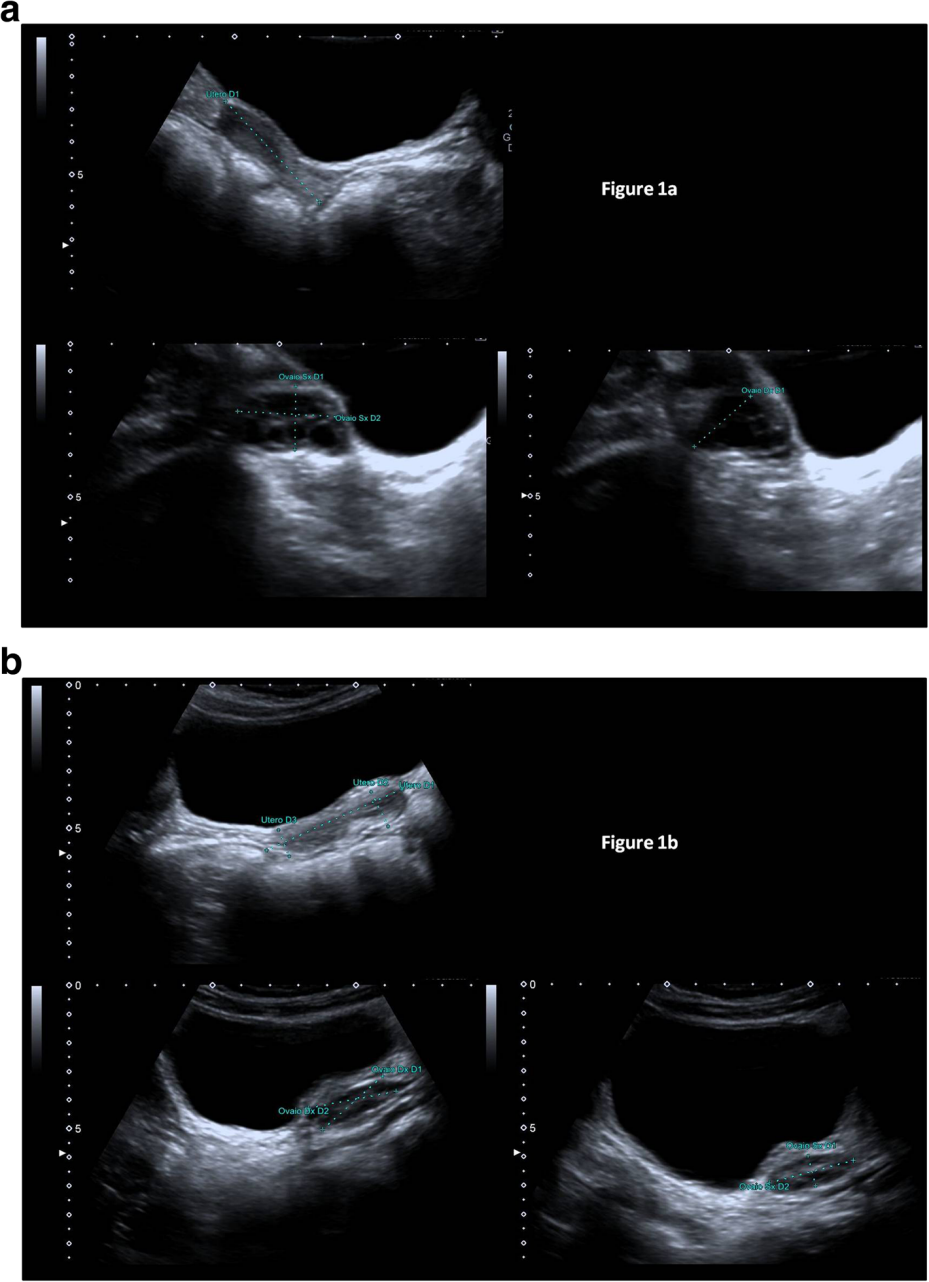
Two girls with precocious puberty diagnosed during lockdown for COVID-19. Patient 1 started puberty at 6.2 years (March 2020): May 2020: height 1.45 SDS, BMI 0.45 SDS; Tanner stage B3 PH2 AH1); patient’s pelvic sonography (May 2020; a). Patient 2 started puberty at 6.9 years (February 2020): May 2020: height 1.36 SDS, BMI 0.52 SDS; Tanner stage B3 PH1 AH1); patient’s pelvic sonography (May 2020; b)
